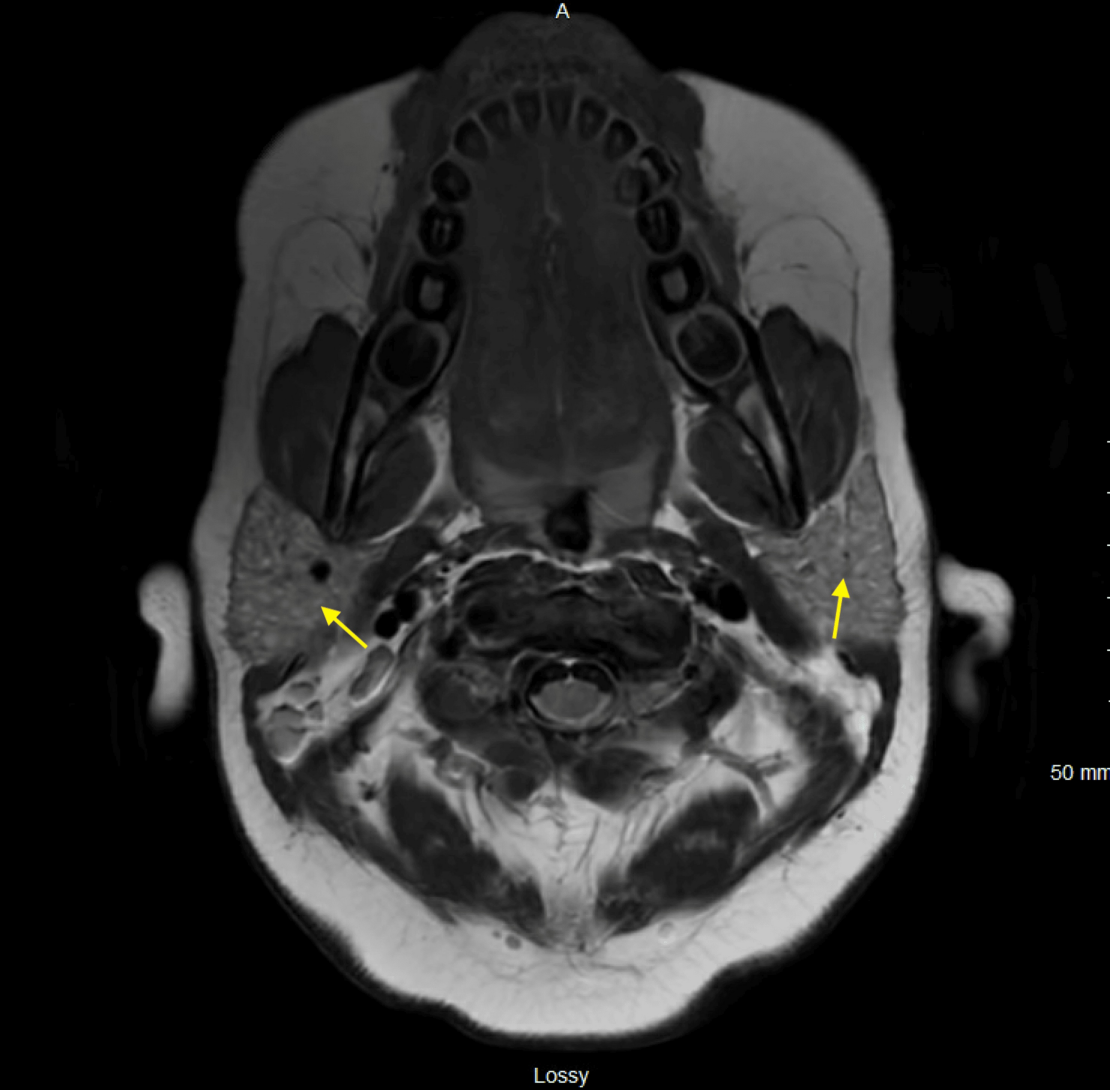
MRI brain w/w/o contrast showing multiple tiny cystic lesions in the bilateral parotid glands suggestive of Sjögren's disease MRI: magnetic resonance imaging
